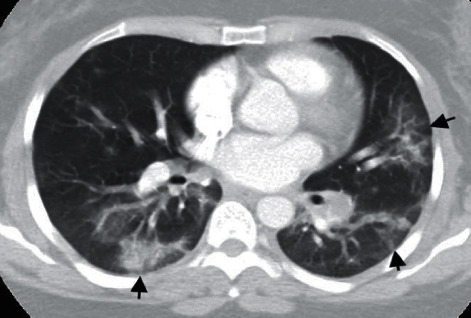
Chest axial CTA image on a lung window shows bilateral multifocal peripheral, lower zonal predominant ground-glass opacities to consolidations (black arrows).
